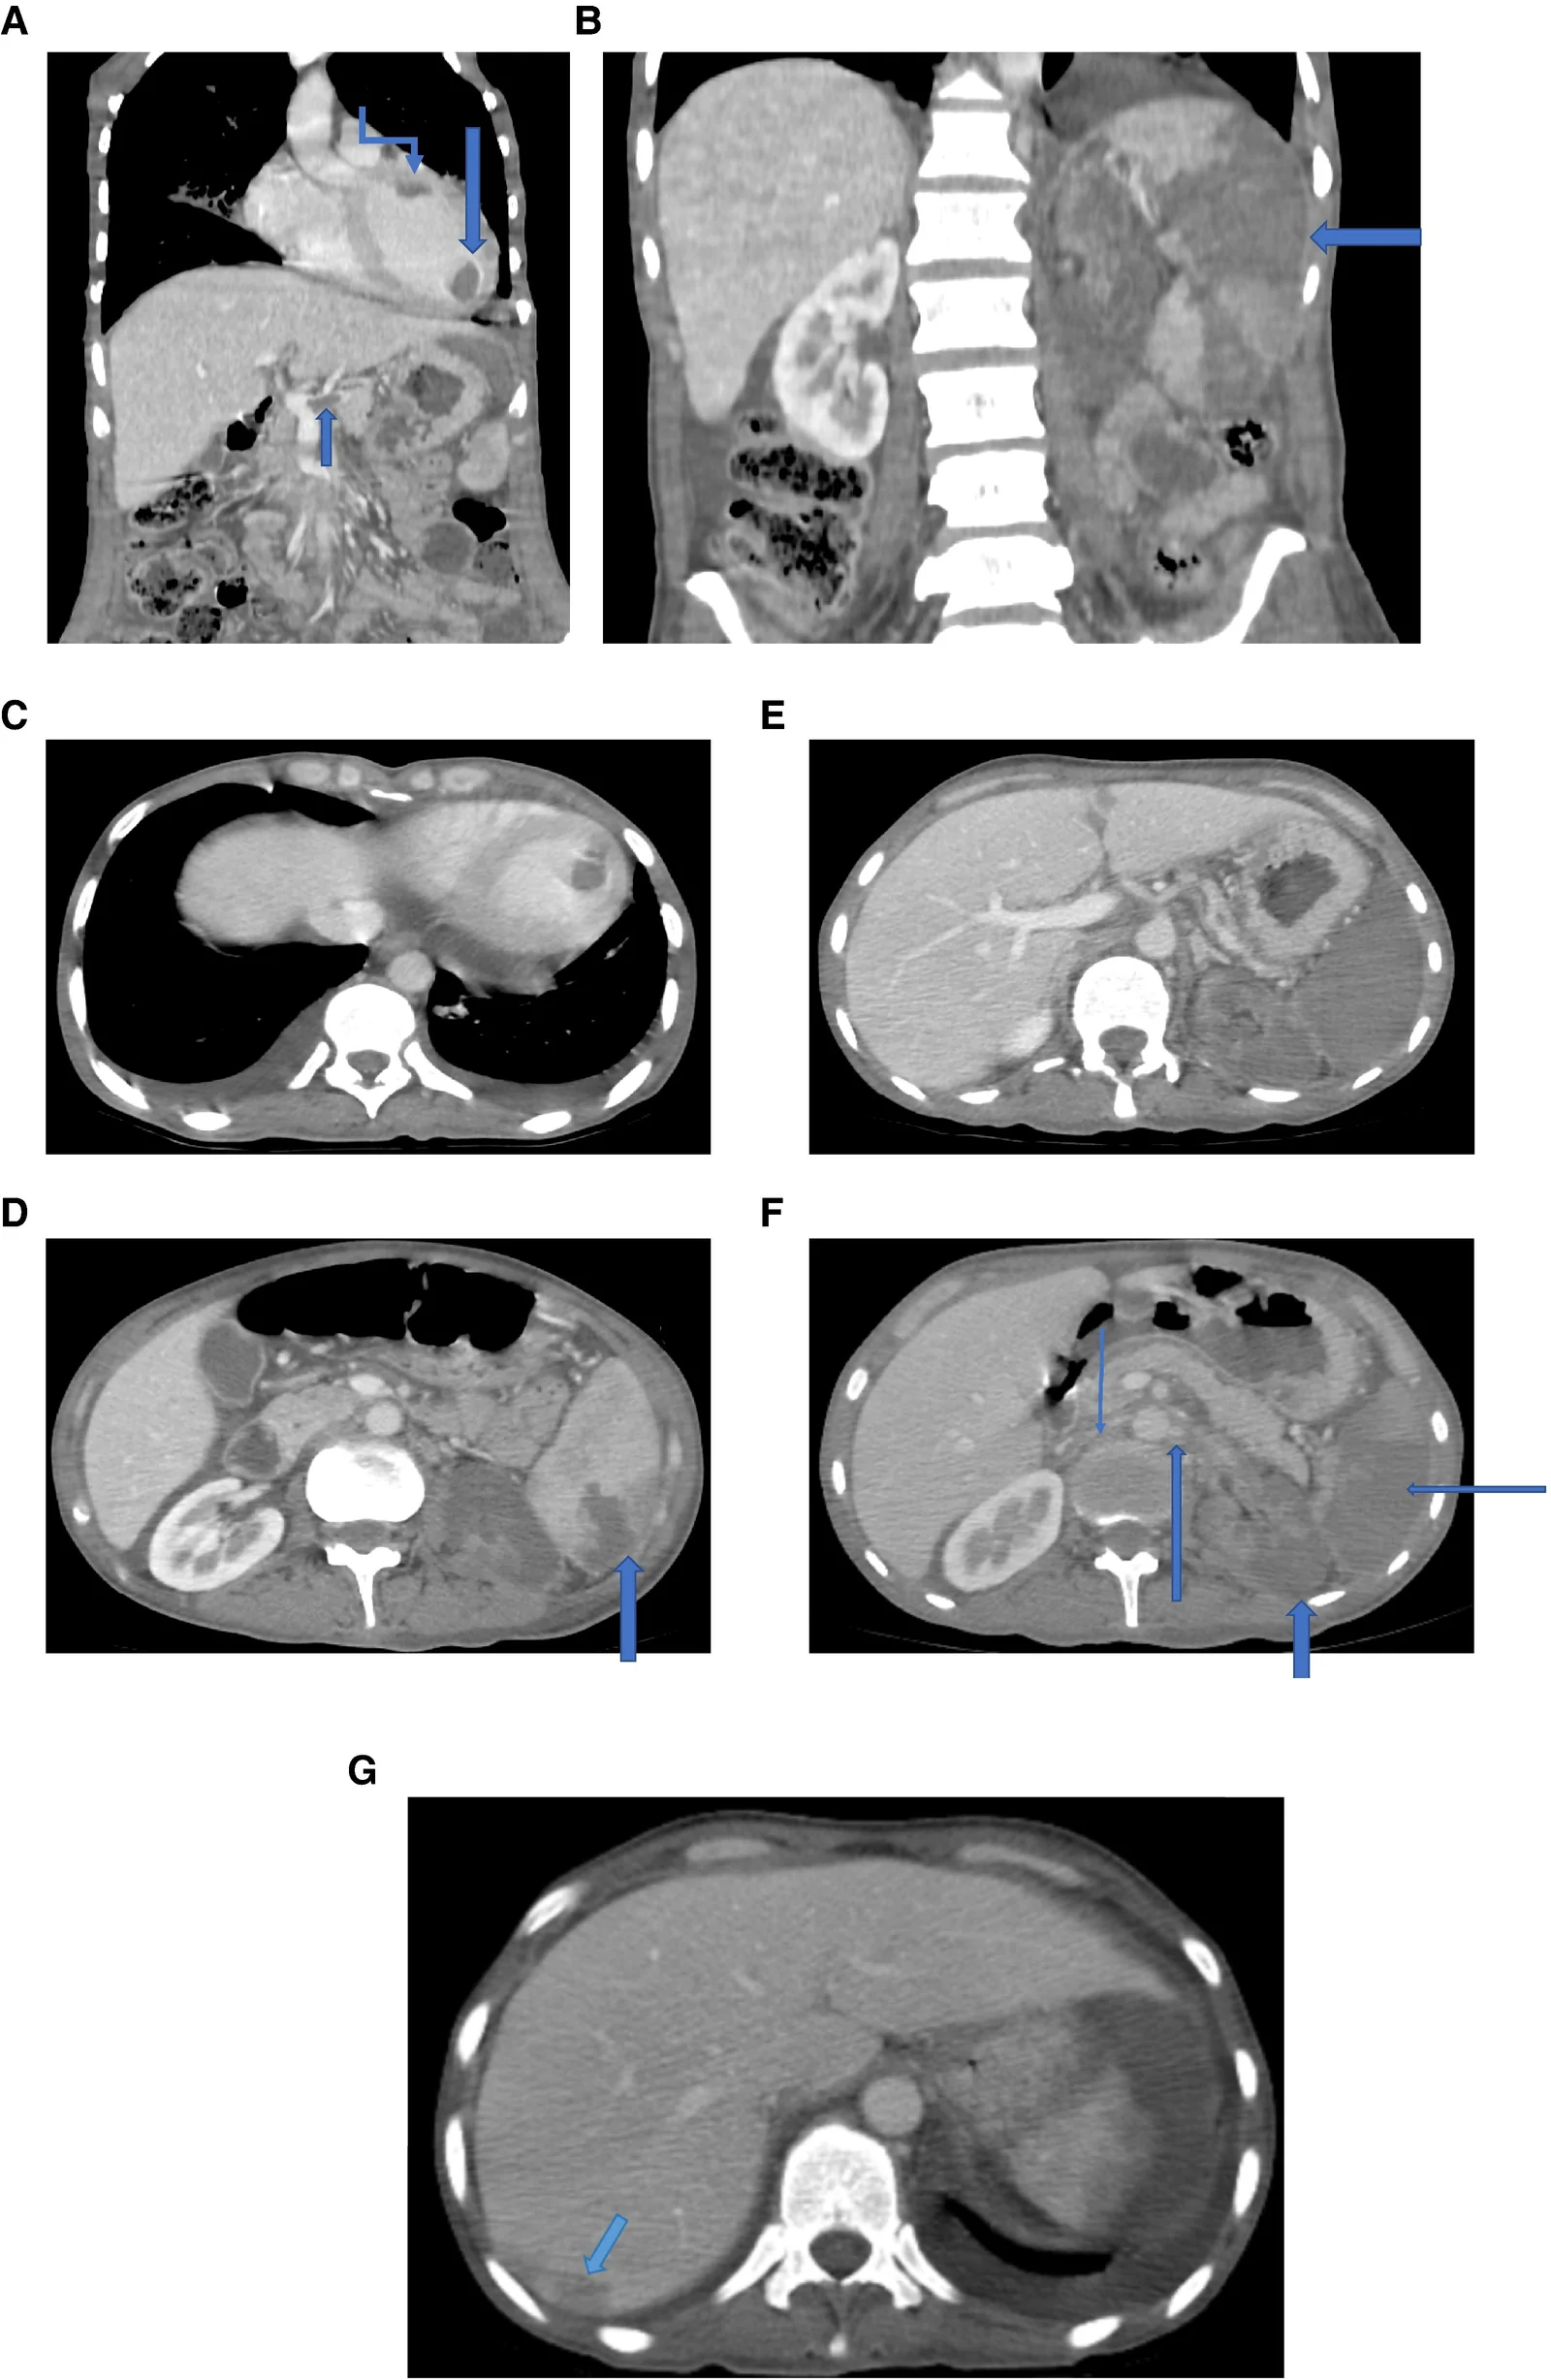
Computed tomography findings at diagnosis. Multiplanar contrast-enhanced thoracoabdominopelvic computed tomography demonstrating intracardiac thrombi and widespread systemic thromboembolic complications. (*A*) Coronal thoracoabdominal computed tomography image demonstrating two intracavitary left ventricular thrombi and splenic vein thrombosis extending from its origin. (*B*) Coronal abdominal computed tomography image demonstrating an enlarged left kidney with multiple peripheral wedge-shaped hypodense lesions, consistent with renal infarctions, with residual enhancing parenchyma after contrast administration. (*C*) Axial thoracic computed tomography image demonstrating a hypodense intracavitary mass within the left ventricular apex, consistent with thrombus. (*D*) Axial abdominal computed tomography image demonstrating near-complete hypodensity of the left renal parenchyma with peripheral capsular enhancement, consistent with extensive renal infarction, with associated splenic infarctions. (*E*) Axial abdominal computed tomography image demonstrating partial splenic vein thrombosis associated with extensive renal and splenic infarctions. (*F*) Axial contrast-enhanced computed tomography image showing bilateral renal and splenic infarctions. The right renal artery appears markedly attenuated, whereas only the ostium of the left renal artery is opacified, with absent opacification of the remaining left renal vascular pedicle. (*G*) Axial contrast-enhanced abdominal computed tomography image demonstrating a peripheral subcapsular hypodense area involving hepatic segments VI and VII, without post-contrast enhancement, consistent with hepatic infarction. Blue arrows indicate the key pathological findings described in panels A, B, D, F, and G.
